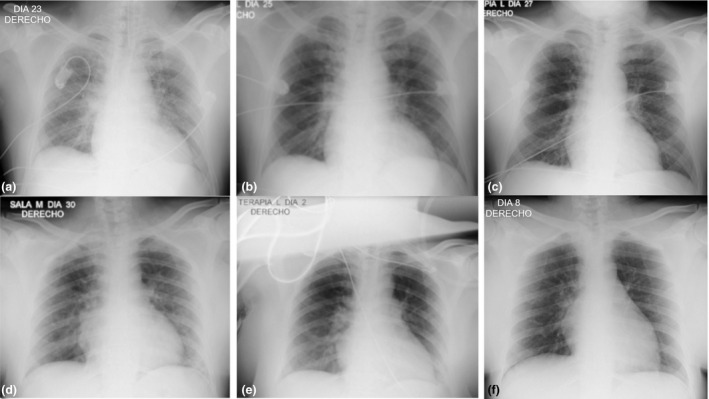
Serial chest radiographs showed significant recovery in 2 COVID‐19 patients after treatment with itolizumab. Patient 1: (a) (Before itolizumab): bilateral hilar vascular thickening, greater on the right side. Right para‐cardiac shadow opacity. (b) (48 h after itolizumab): decreased bilateral hilar vascular thickening. Decreased opacity in the right para‐cardiac shadow and thickening of the right basal hilum. No pleuro‐pulmonary lesions. (c) (5 days after itolizumab): favorable radiological evolution, with disappearance of bilateral hilar vascular thickening and the right para‐cardiac opacity. No pleuro‐pulmonary lesions. Patient 2: (d) (Before itolizumab): diffuse veil opacities that project in the right para‐cardiac region and in the lower left lung field. (e) (48 h after itolizumab): decrease in veil opacities. (f) (8 days after itolizumab): no pleuro‐pulmonary lesions. Favorable radiological evolution, with disappearance of the lung lesions.
